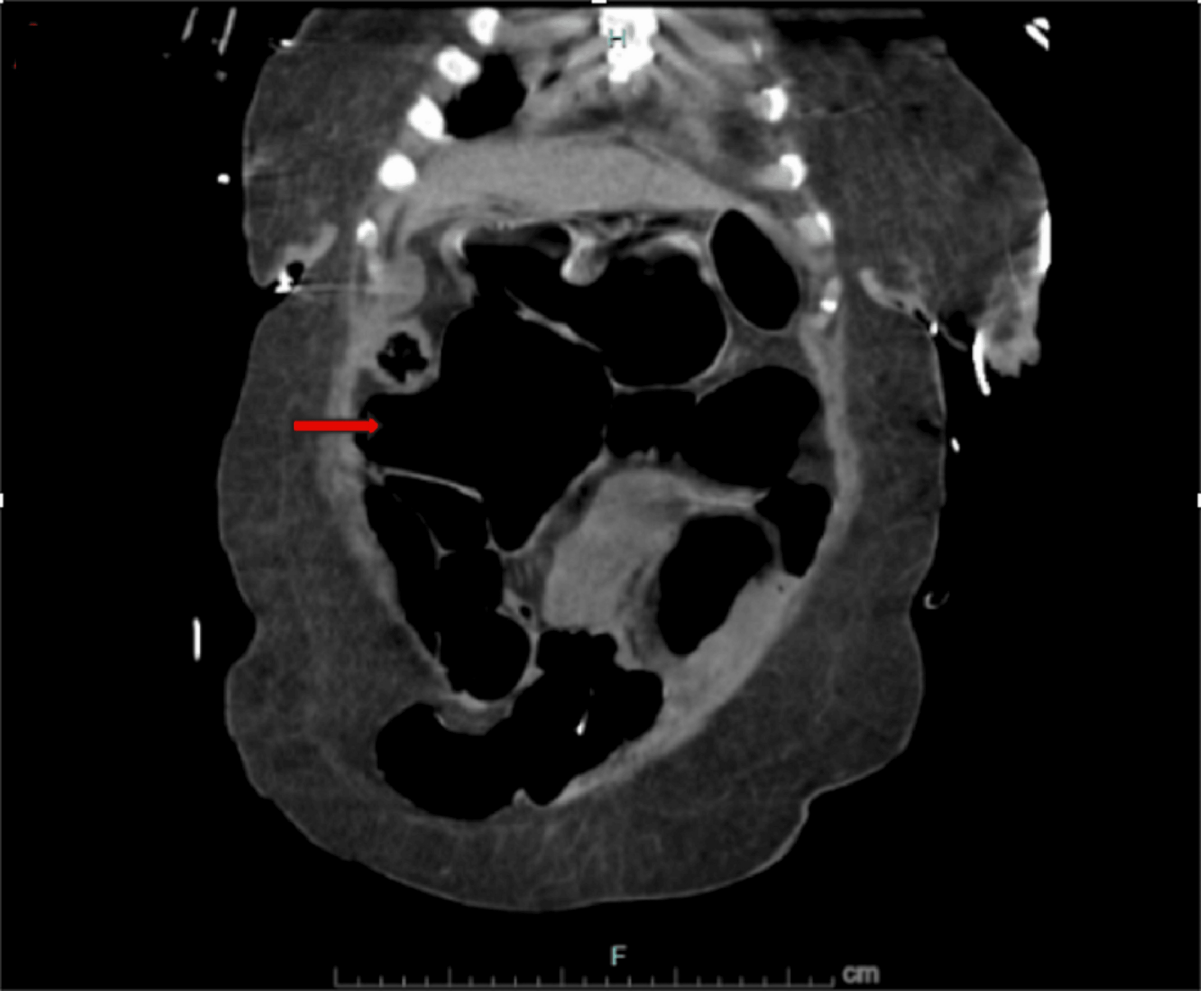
Coronal CT scan of the abdomen and pelvis without contrast. Air-fluid levels (arrow) suggestive of intestinal obstruction.
